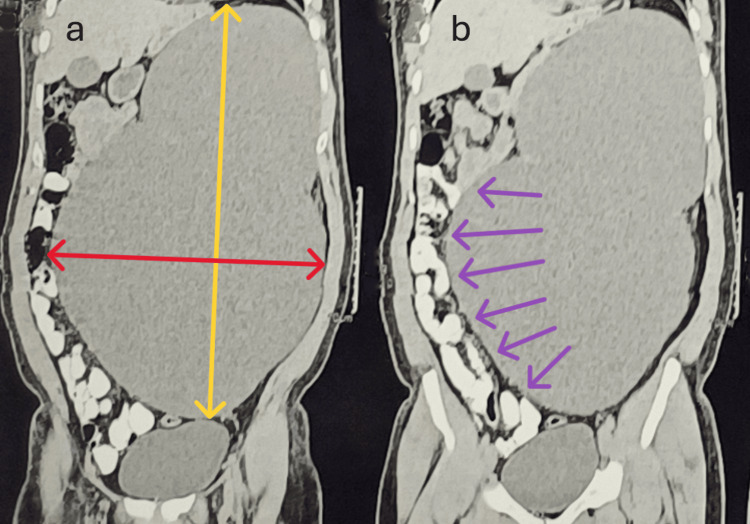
Coronal sections of CT. (a) Vertical (marked by a bidirectional yellow line) and horizontal (marked by a bidirectional red line) extent of the parapelvic cyst. (b) The displacement of the bowel loops to the contralateral hemiabdomen (marked by purple arrows) caused by the cyst wall.
